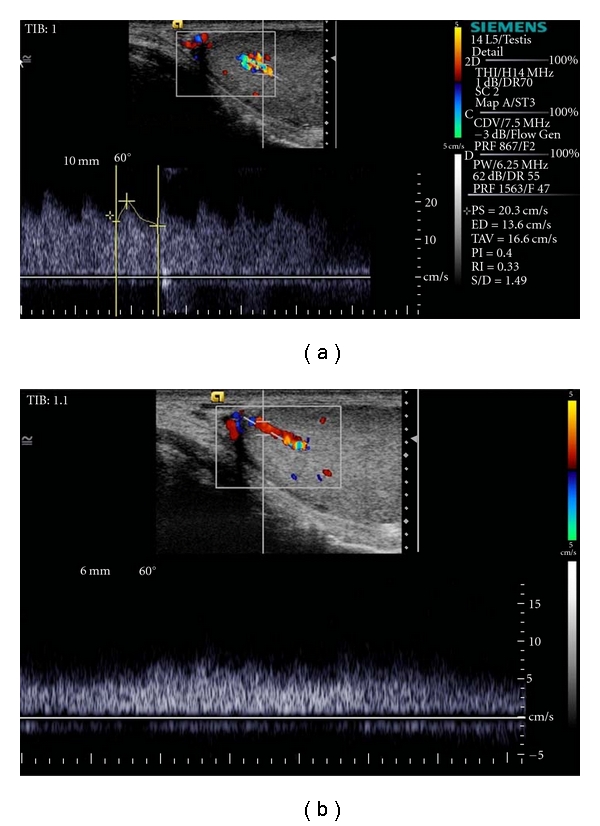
Spectral Doppler ultrasound depicts arterial flow in the feeding pedicle (a) and pulsatile venous flow in the drainage vessel (b).
